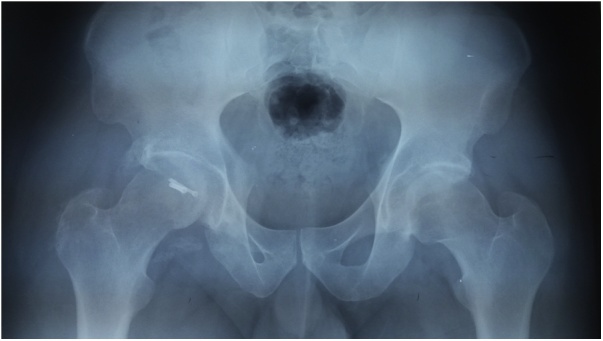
two years pelvic post-operative X-ray showing absence of osteoarthritis or avascular necrosis signs, but a right hip non-bridging Brooker type I heterotopic ossification was noticed.
